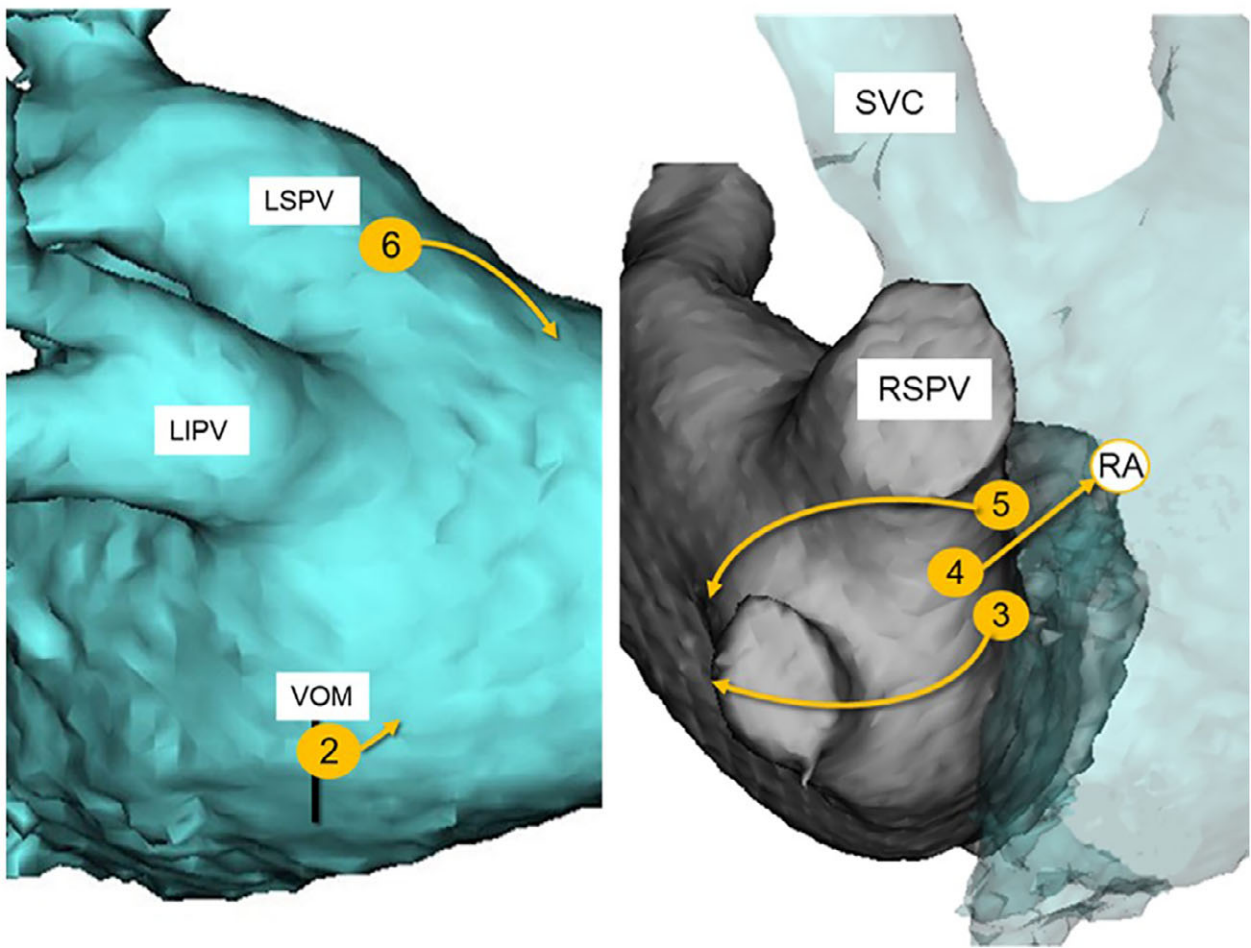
The origins of each Epi‐AT were marked on CT images (number in circle). Arrow heads indicated each earliest activation sites in endocardial activation maps. LIPV: left inferior pulmonary vein, LSPV: left superior pulmonary vein, RSPV: right superior pulmonary vein, SVC: superior vena cava, VOM: the vein of Marshall
